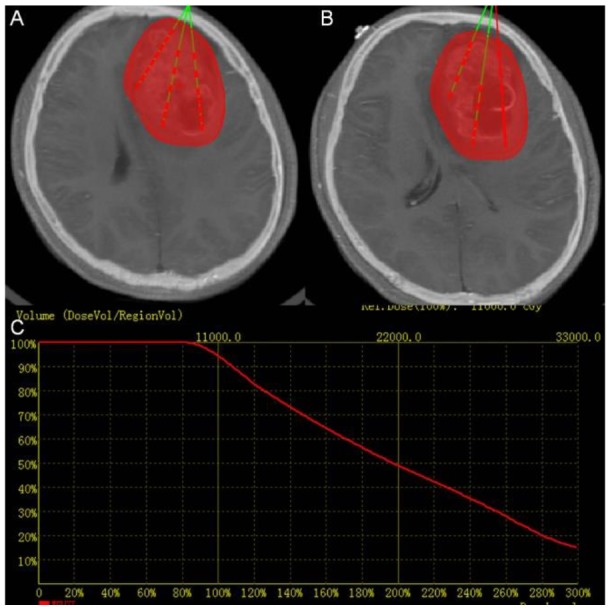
Treatment planning (A) and (B) Two slices of CT-MRI fused images showing the PTV in red and planned catheter and seed placement in green (C) Dose volume histogram of PTV of pre-operation treatment planning. The red curve shows the dose volume histogram of the target.
